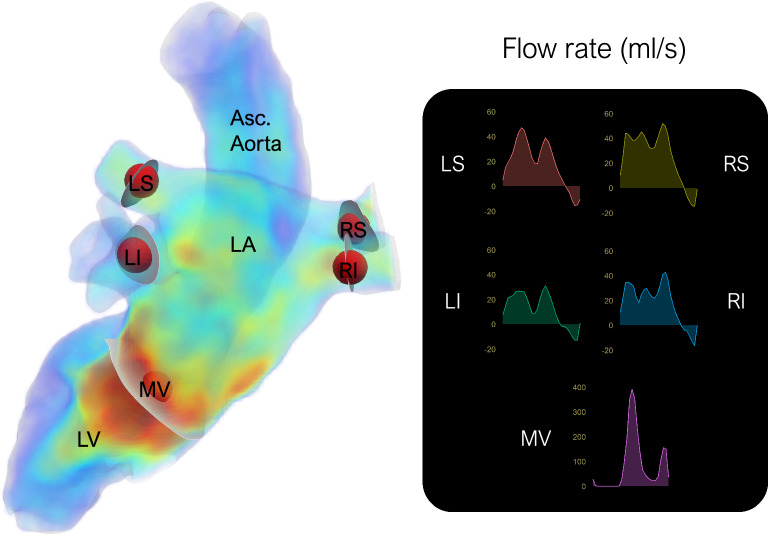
A volumetric rendering of the 4D flow magnetic resonance imaging velocity field in the left heart is shown, encompassing the left atrium, left ventricle, and ascending aorta. During processing, spheres are manually placed within regions of interest to serve as sample volumes to determine the dominant flow direction and define vessel cross-sections (shown in grey) for flow rate computations. On the right, the corresponding flow rates (*ml*/*s*) are shown for the mitral valve and the four pulmonary veins. LA: Left atrium, LV: Left ventricle, Asc. Aorta: Ascending aorta, MV: Mitral valve, RS: Right superior, LS: Left superior, RI: Right inferior, LI: Left inferior. Images created using ParaView (version 5.13.3).
